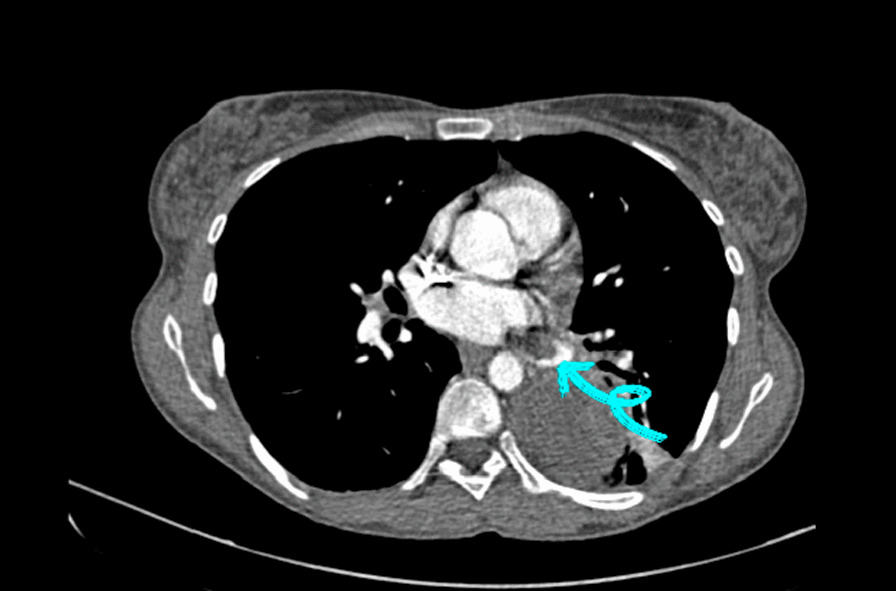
Mediastinal view of the chest computed tomography angiography: blue arrow shows the thrombus in the left infero-posterior pulmonary vein
